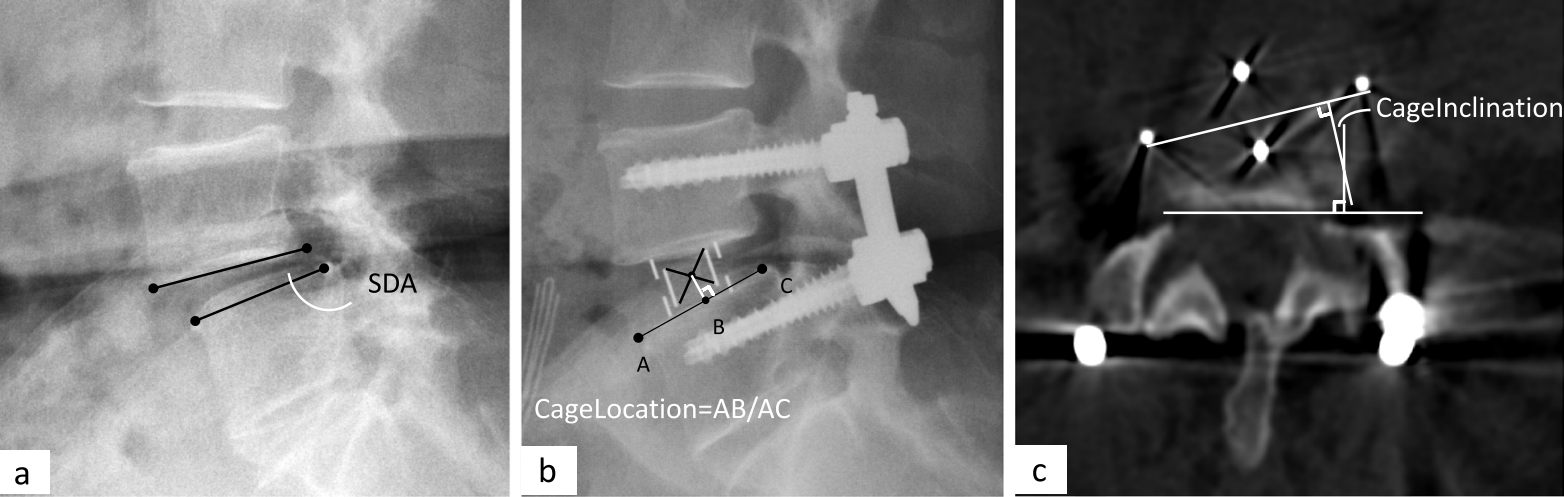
Definitions of radiographic parameters. a Segmental disc angle (SDA) on the standing lateral view of lumbar spine. b CageLocation, which is the ratio of AB to AC. B is the intersection point between perpendicular line from the midpoint of cage and the upper endplate. A and C are the anterior and posterior margin of upper endplate. c CageInclination, which is the angulation between axis of cage and posterior border of vertebral body
